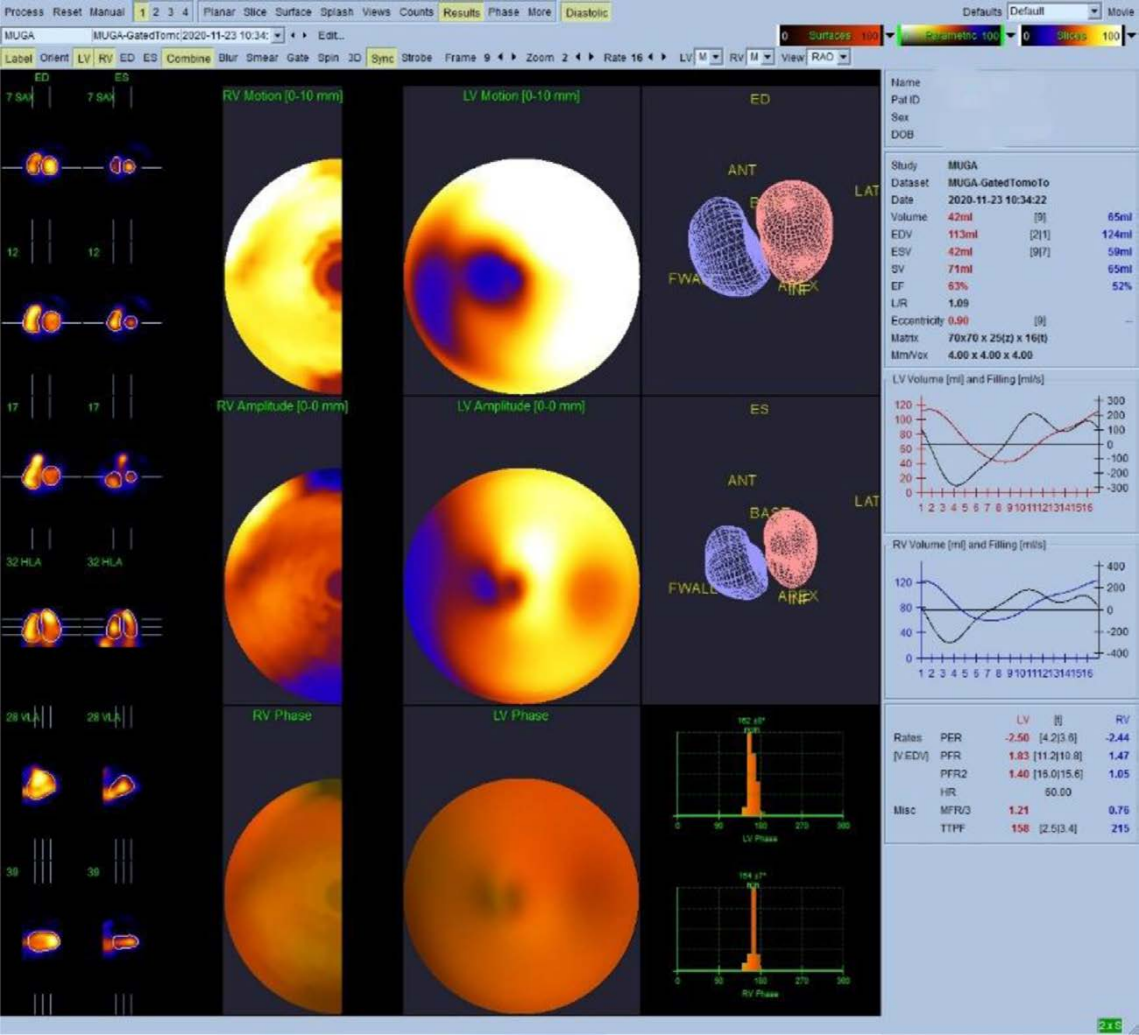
CZT-SPECT tomographic image and parameters calculated by Cedars-Sinai G-BPGs.
